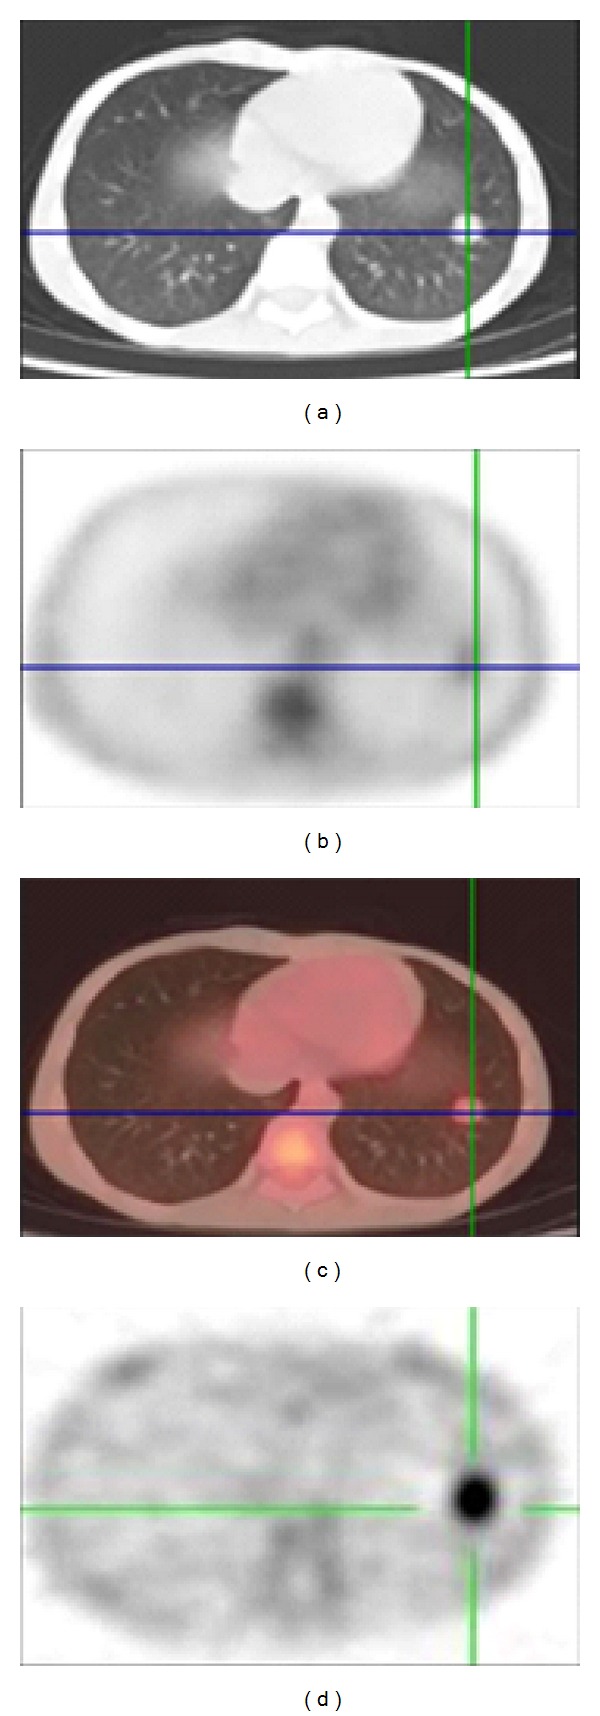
Showing the intense uptake in the left lung lower lobe nodule with higher intensity of uptake on ^99m^Tc-DMSA (V) (d) than [^18^F]FDG.
